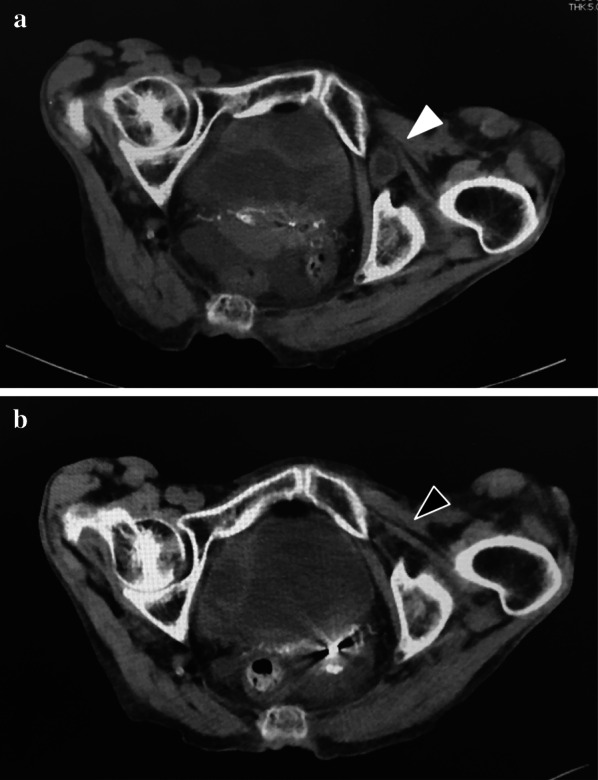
a CT image showed incarcerated obturator hernia. (White arrow head). b CT image showed disappeared incarcerated obturator hernia. (black blank arrow heads)
